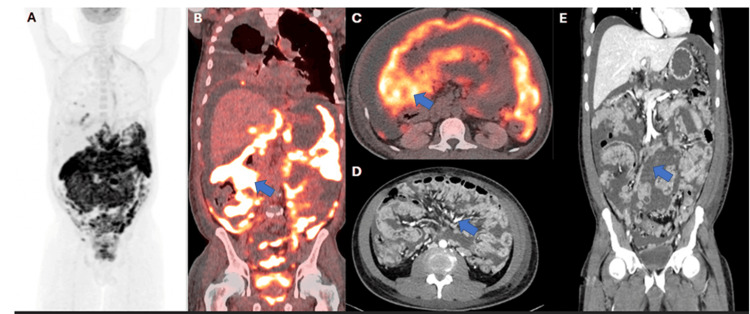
PET/CT imaging (A) Maximum intensity projection (MIP) 18F-FDG PET image. ​(B, C) Coronal and axial planes of PET/CT images demonstrate extensive FDG uptake throughout the peritoneum and mesentery, consistent with peritoneal lymphomatosis (see arrows).​ (D, E) Contrast-enhanced CT of the abdomen and pelvis from 10 days prior to the PET/CT, in axial and coronal planes, demonstrates large-volume ascites with diffuse peritoneal and omental implants (see arrows).​ 18F-FDG: fluorine-18 fluorodeoxyglucose
